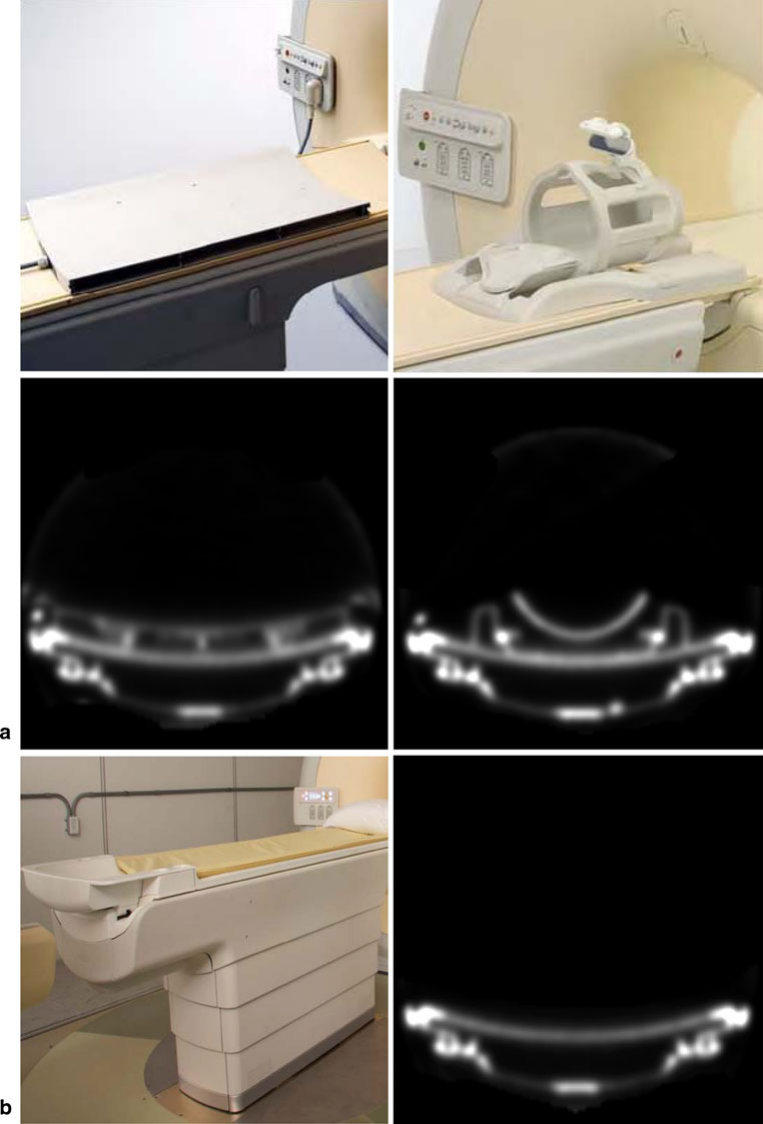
In PET/MR, coils and other MR devices not visible in the MR images must be generated as template images, for example, in PET transmission scans and added to the attenuation map. Examples of a spine and a head coil (a) and a patient table (b) as well as one transaxial slice of the corresponding templates generated from PET transmission scans are shown [54] (courtesy of B. Zhang)
